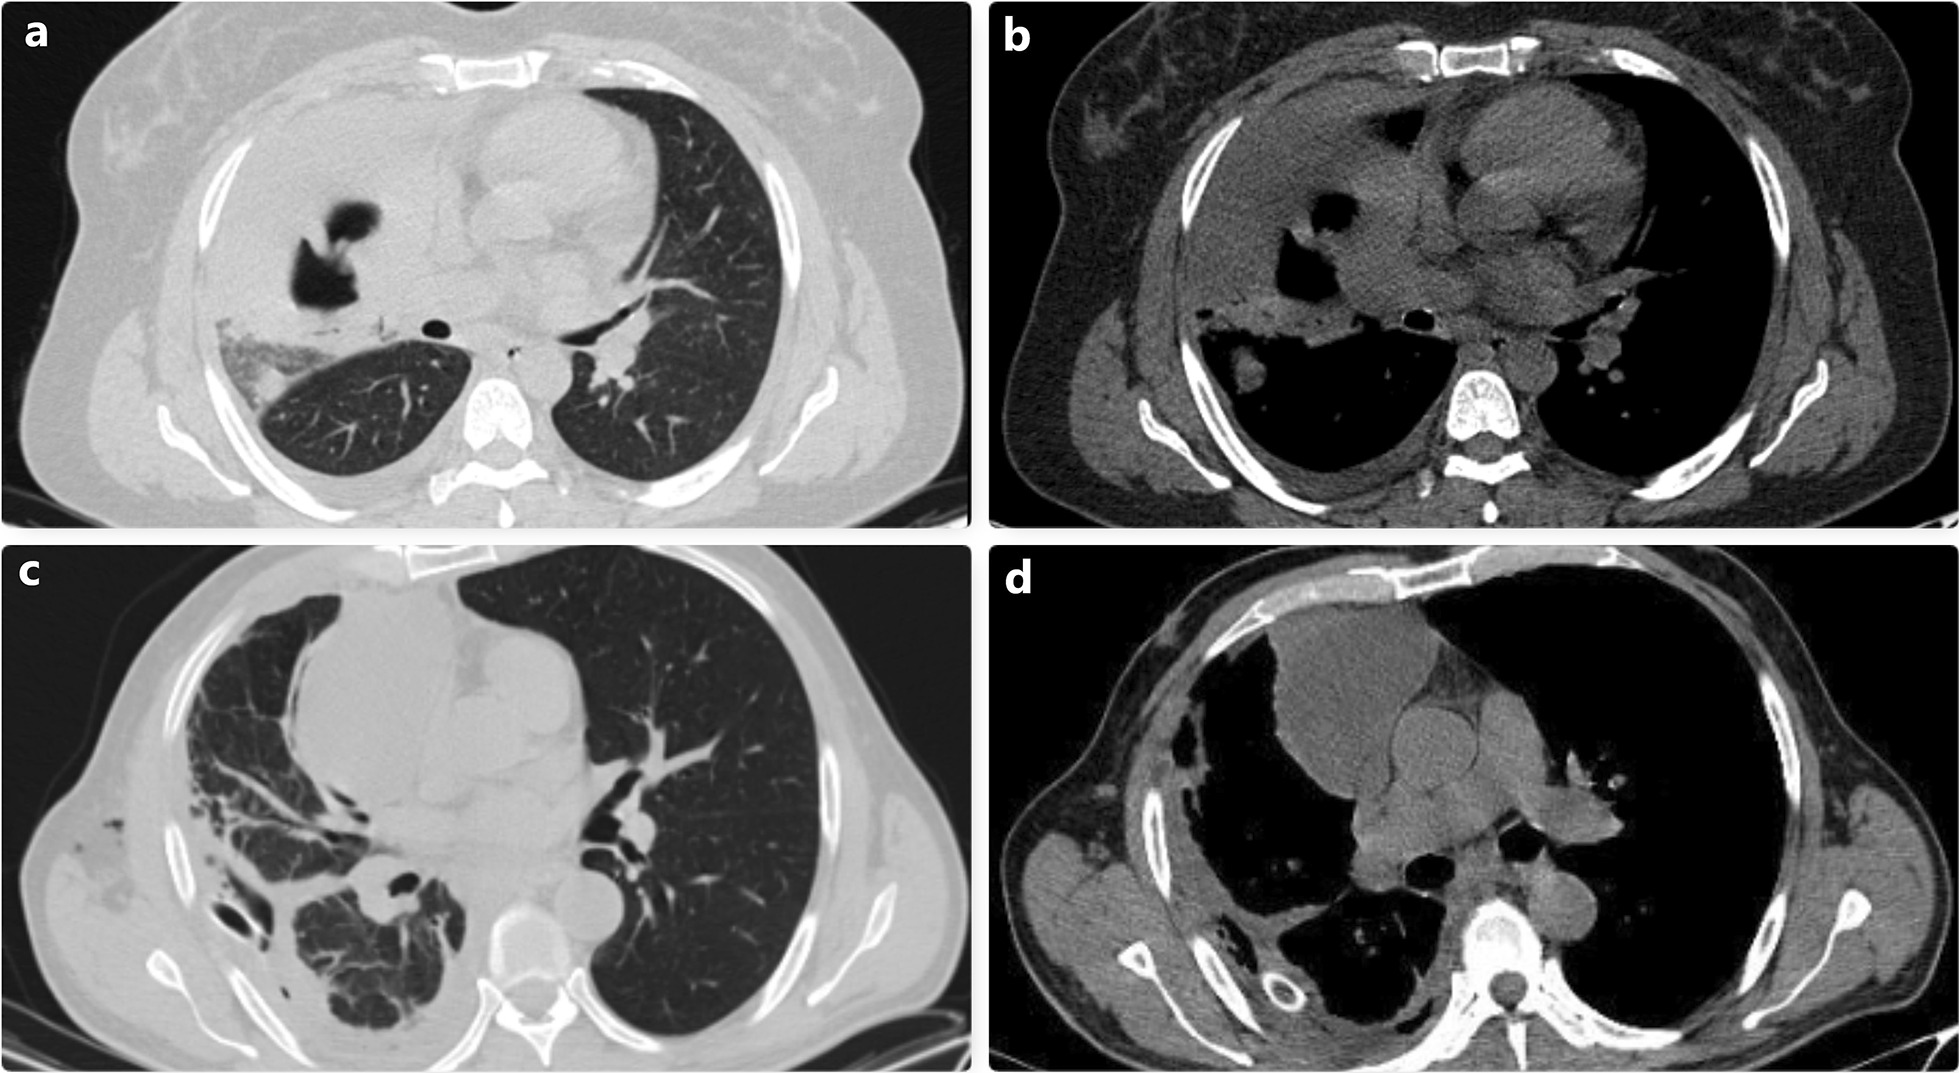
Thoracic CT images of two different patients who were followed up with the diagnosis of empyema are shown. The operation performed due to the persistence of the infection status and loculation despite medical treatment revealed that these cases had complicated hydatid cysts
